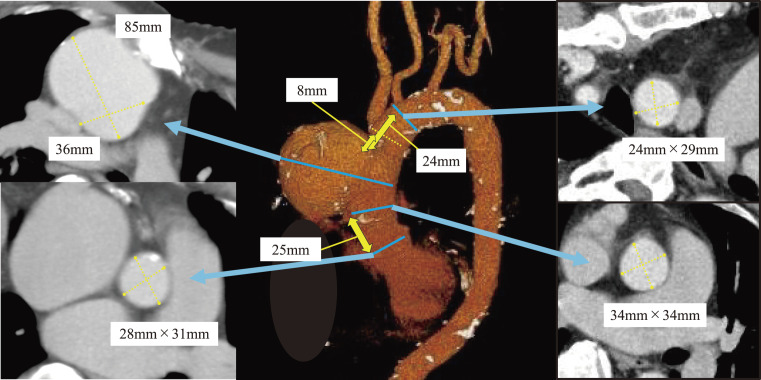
Preoperative CT. A pseudoaneurysm of the ascending aorta measuring 85 × 65 mm was identified. The distance from the STJ to the proximal edge of the pseudoaneurysm was 25 mm, while the distances from the BCA and LCCA to the lesion were 8 and 24 mm, respectively. The aortic diameters at the proximal and distal sealing zones were 34 and 28 mm, respectively, and the aortic wall characteristics were deemed favorable. CT: computed tomography; STJ: sinotubular junction; BCA: brachiocephalic artery; LCCA: left common carotid artery
